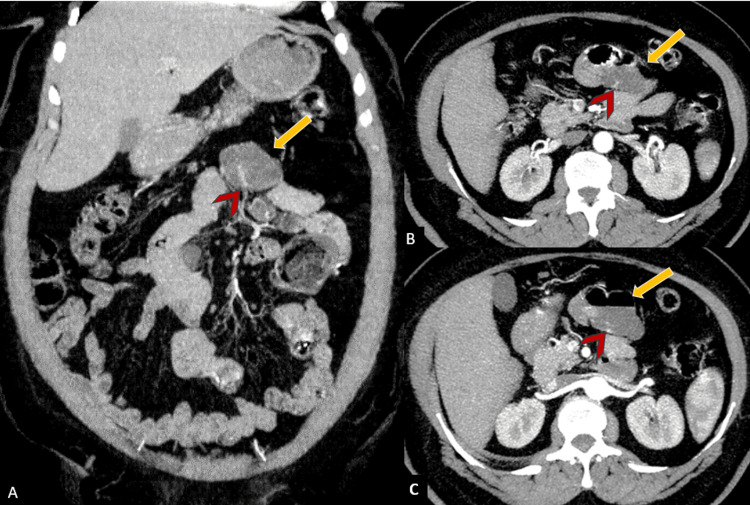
Arterial phase CT angiography with coronal (A) and axial (B, C) images showing prominent dilated vascular structures (red arrowheads) within the posterior wall of the proximal jejunal diverticulum (yellow arrow).
